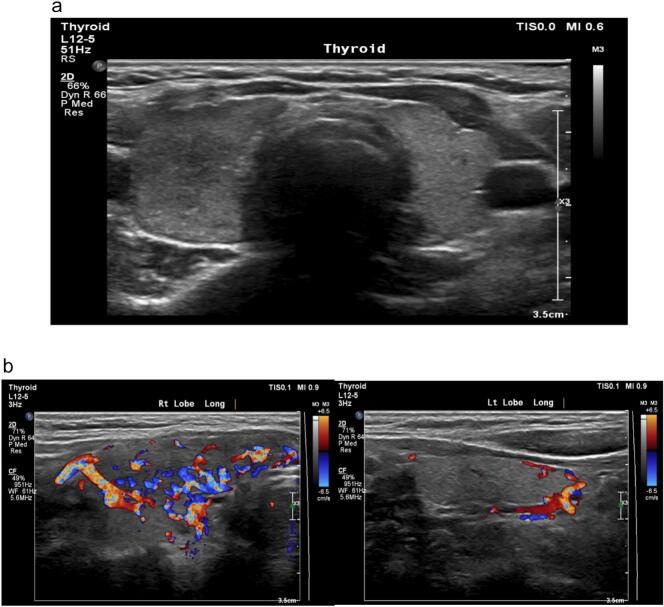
a. Neck ultrasound showing enlarged right thyroid lobe. b. Color doppler neck ultrasound demonstrating the greater hypervascularity of right thyroid lobe.
